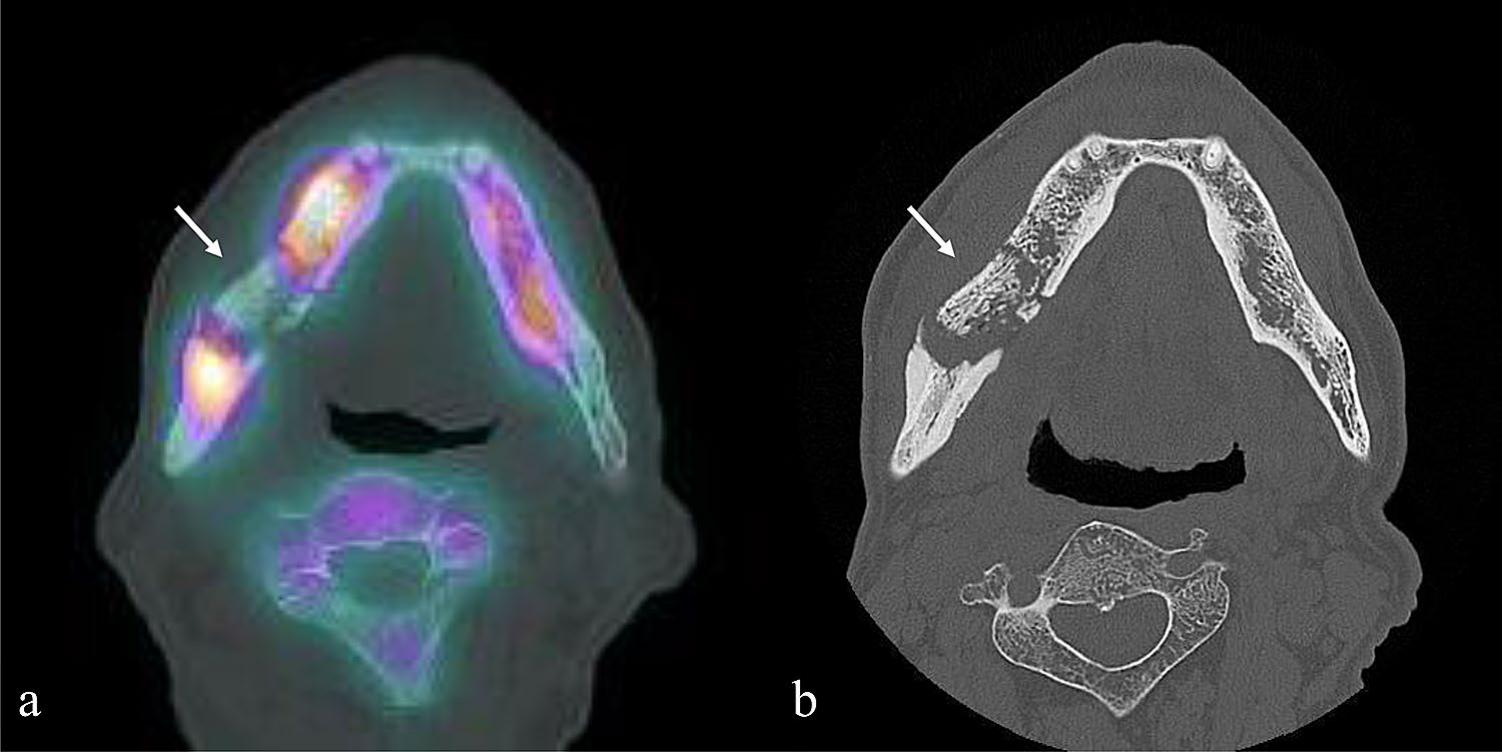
Findings of a “cold-in-hot” region. a SPECT/CT image showing an involucrum with low accumulation surrounded by high-accumulation area on the right mandible (see arrow). b CT image showing that the involucrum is separated from the surrounding bone. *CT* computed tomography, *SPECT* single photon emission computed tomography
